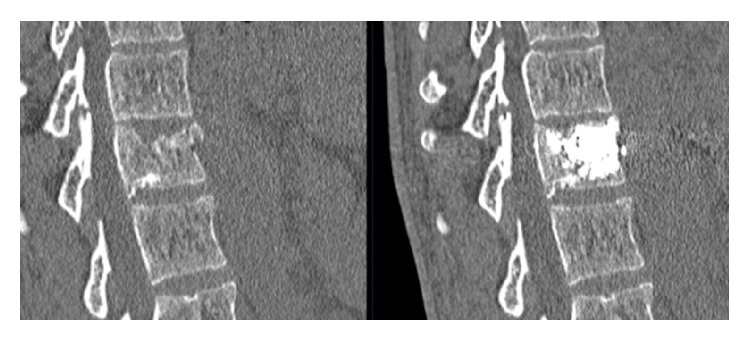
Preop and postop CT scan of the fractured vertebra.
